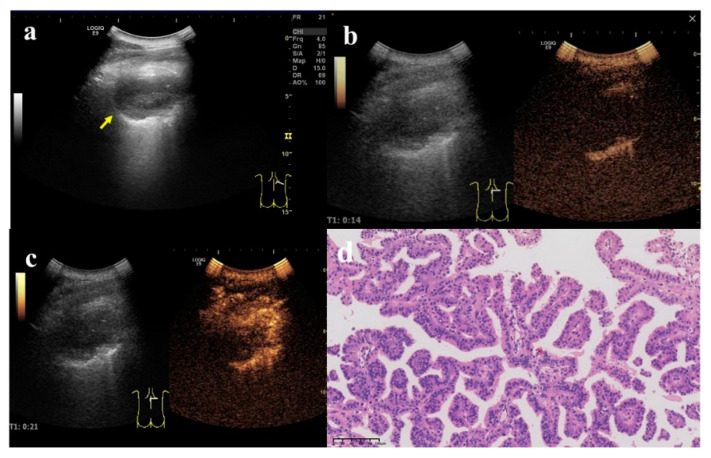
A 58-year-old male with a lesion occupying the deep space in the lower corner of the right shoulder armor. (a) Two-dimensional ultrasound showed a hypoechoic nodule, size 5.5 × 4.5 cm (arrow). At least one angle (between lesion border and thoracic wall) was obtuse. (b) Contrast-enhanced ultrasound showed that the enhancement time of lung tissue was 10 s and that of nodule was 14 s. (c) The enhancement began from the periphery to the center. The enhancement reached the peak in 30 s, and the enhancement degree was obviously uneven. (d) Pathologic evaluations revealed lung adenocarcinoma, mainly solid and acinar (×200).
